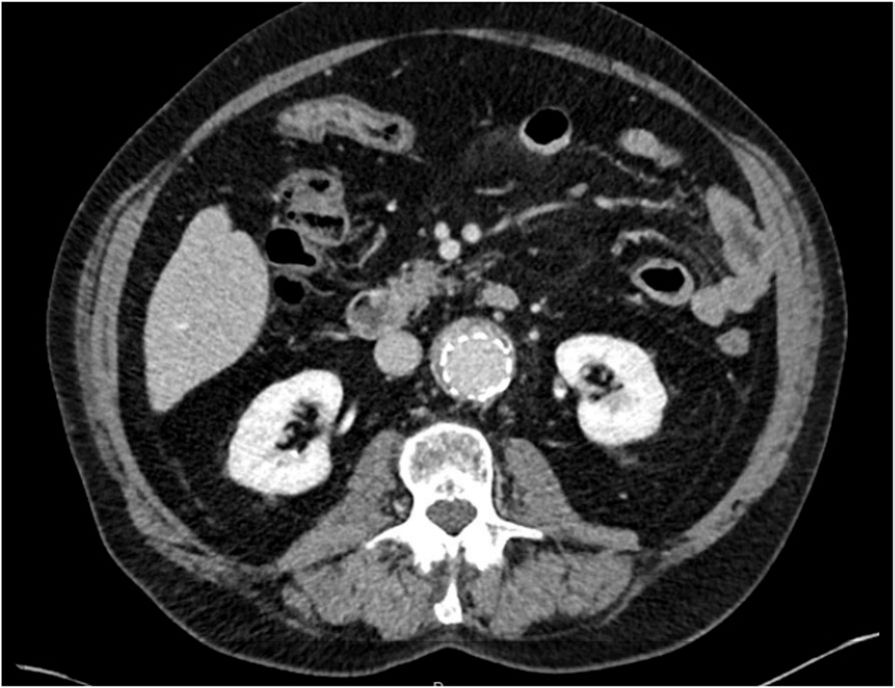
Patients Computed Tomography Angiography showing a type 1 endoleak
